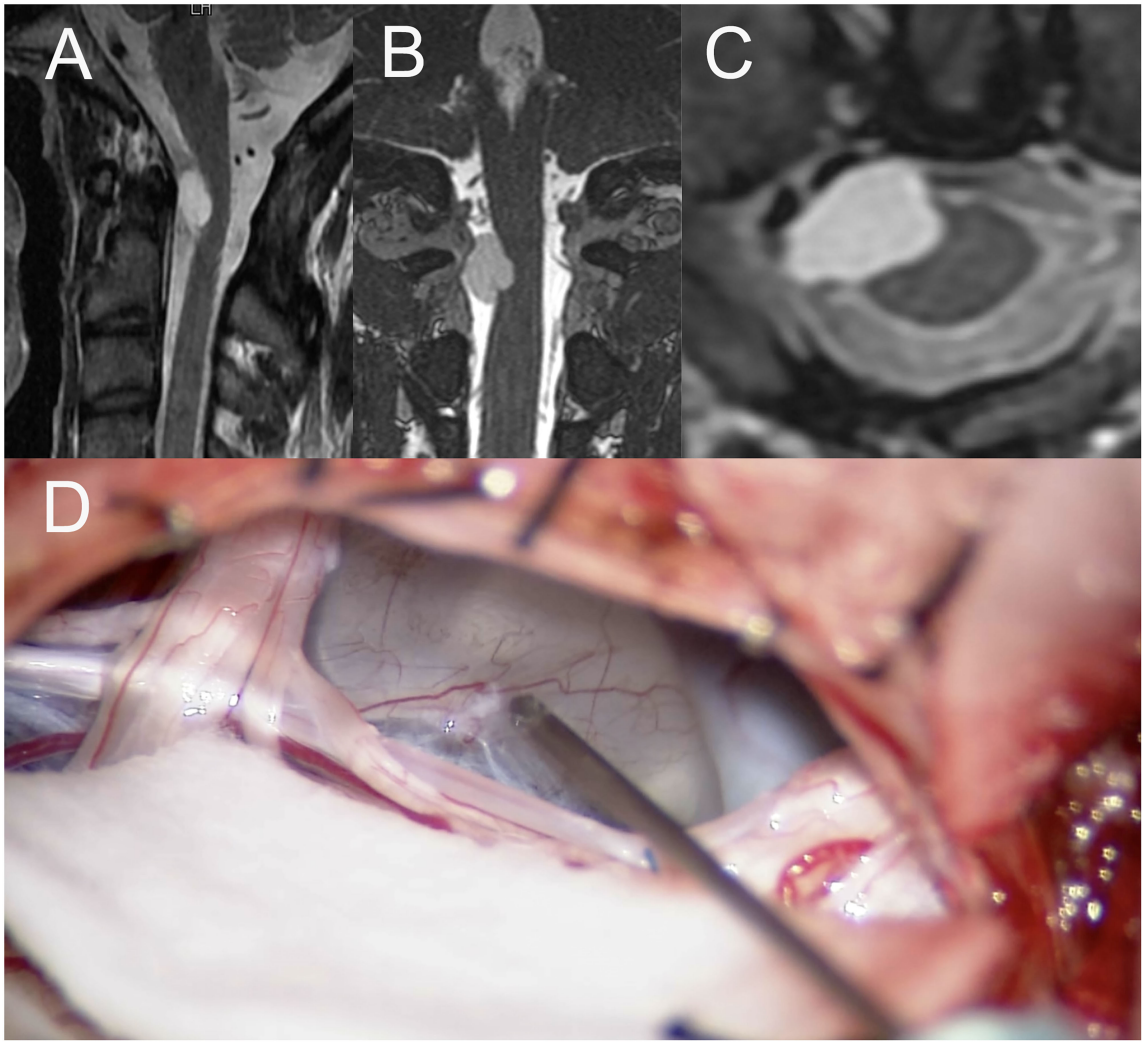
(A) Sagittal image of preoperative T1WI of MRI, (B) the coronal image of preoperative T1WI of MRI, (C) the axial image of preoperative Gd-enhanced T1WI of MRI, (D) intraoperative photograph of tumor resection by posterior approach. TIWI, T1-weighted image; MRI, magnetic resonance imaging; T2WI, T2-weighted image, Gd, gadolinium.
